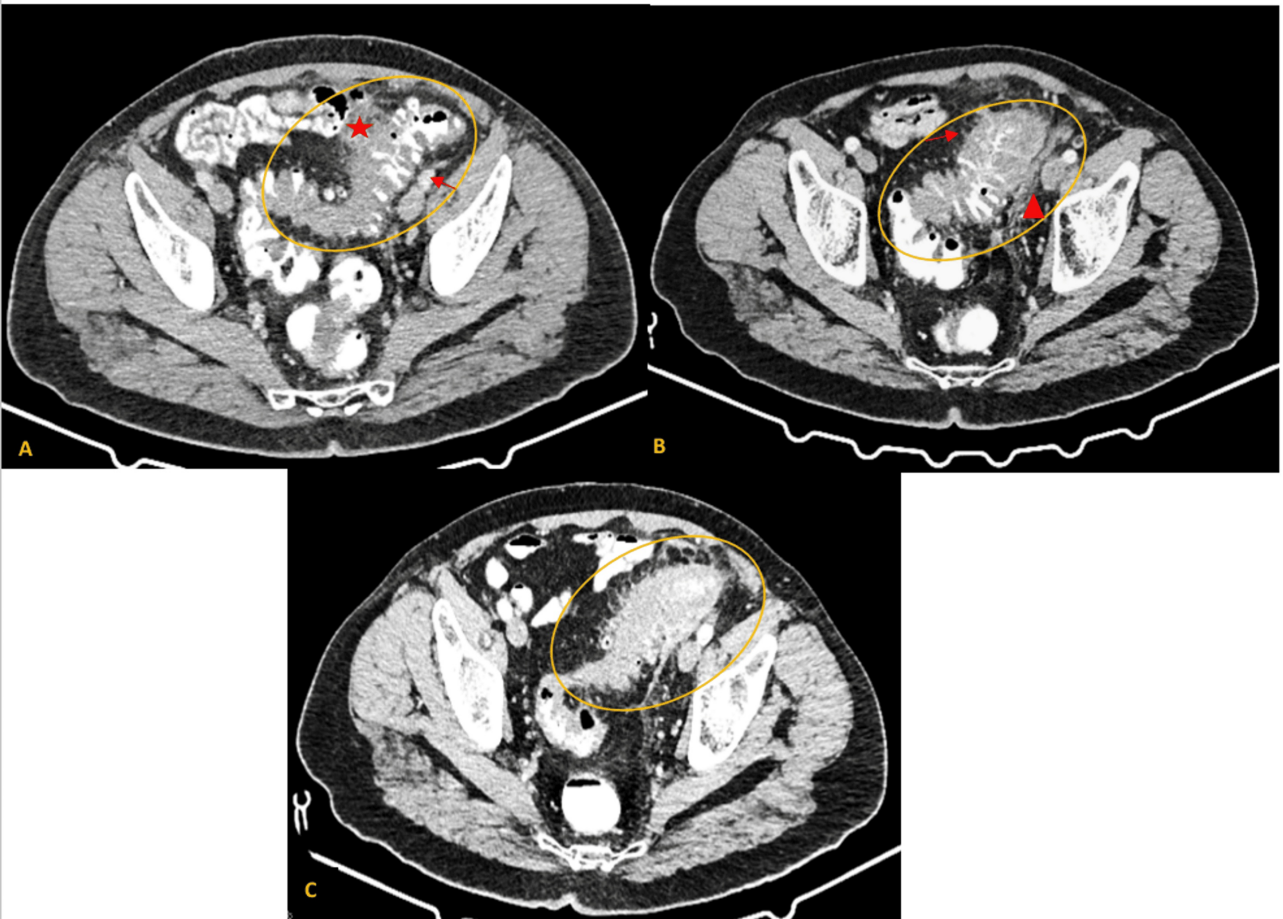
A case of 55-year-old male with two episodes of diverticulitis associated with multiple diverticula present in the sigmoid colon (Α) First episode: The sigmoid colon appears with wall multiple diverticula (yellow eclipse), wall thickening (red asterisk), and micro-fluid collections located in the left iliac fossa (red arrow) classified as Hinchey Ia. (B) Recurrent episode two years after the initial diagnosis: The middle part of the sigmoid colon appears with augmented wall thickness (yellow eclipse), multiple diverticula (red arrow), and pericolic fat stranded (red triangle) classified as Hinchey Ia. (C) Outcome after five days of conservative treatment as seen in a new CT scan: Colonic wall and pericolic fat inflammation seem to have subsided (yellow eclipse).
